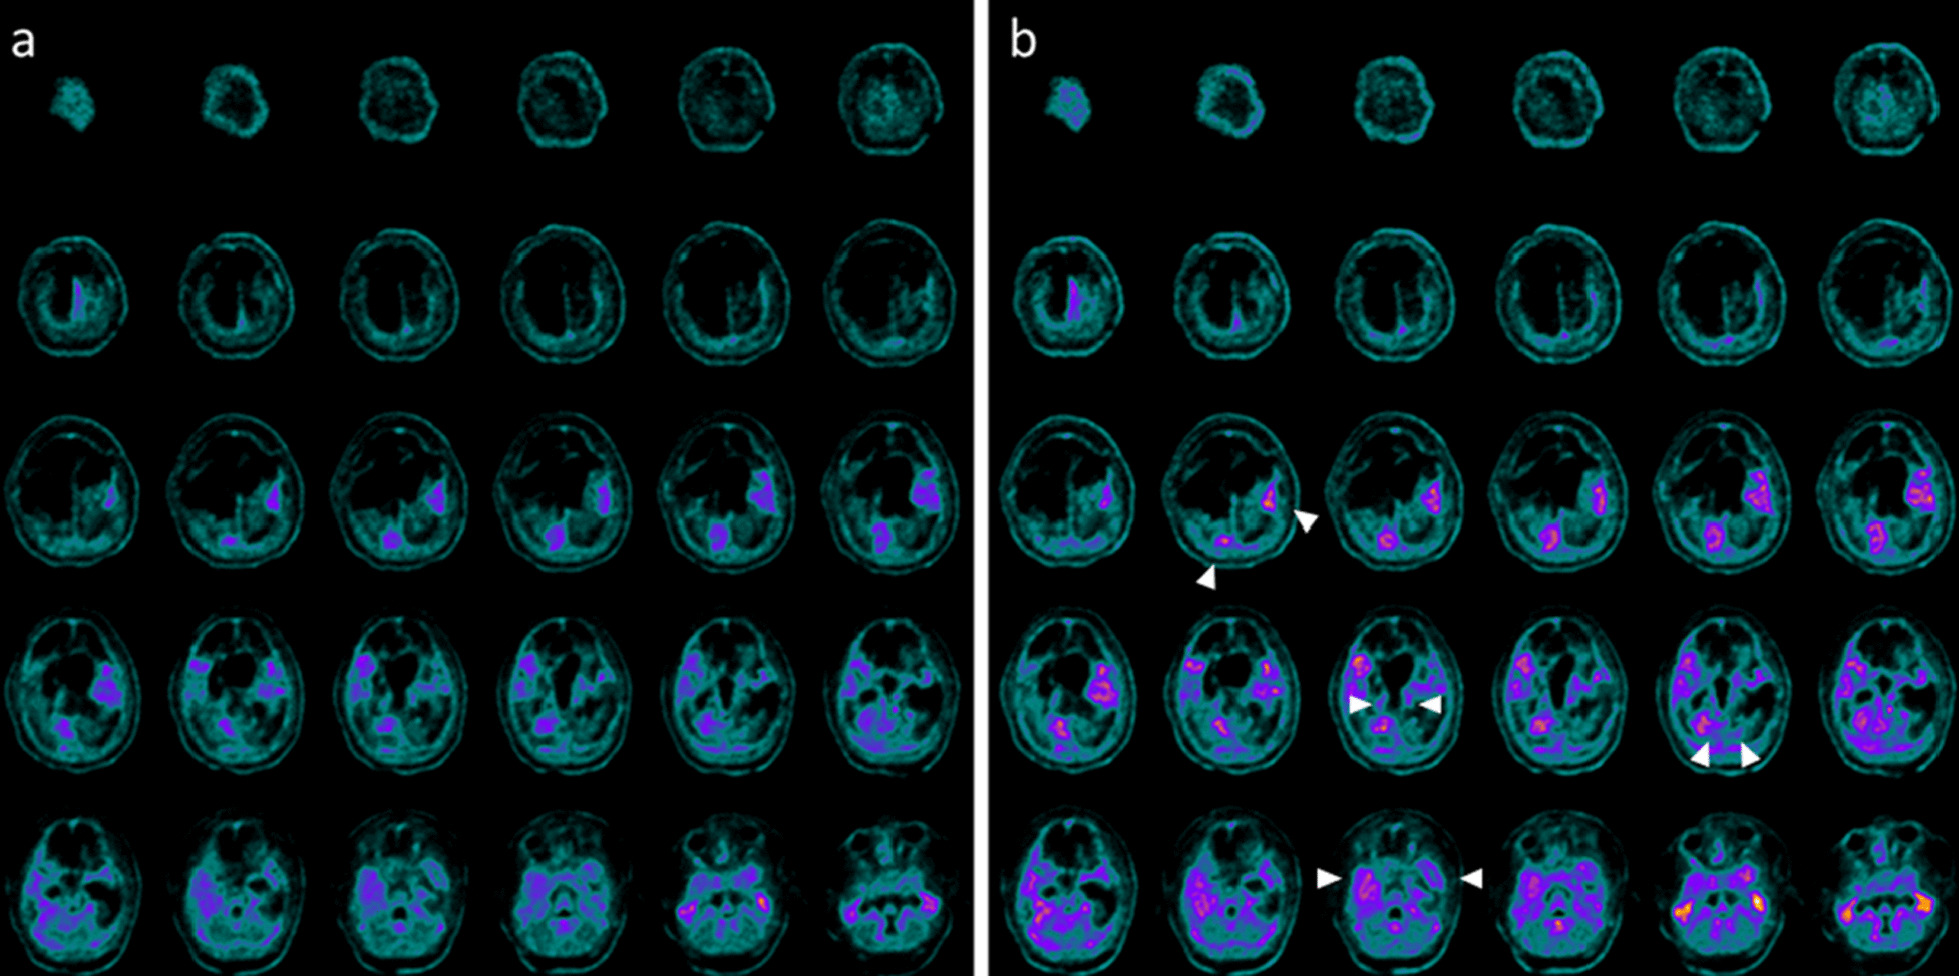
PET before and after the treatment with hr-NGF. a, b: Brain 18F-FDG PET axial slices performed before (a) and after b hr-NGF treatment. A severe global reduction in 18F-FDG uptake was observed in all cortical and subcortical regions as well as in the cerebellum (a). After hr-NGF administration, an increase in radiotracer uptake was detected in the left and right temporal cortex (right: + 18%; left: + 15%), bilateral parietal cortex (right: + 18%; left + 15%), right and left occipital cortex (right: + 10%; left: + 13%) and right and left thalamus (+ 7% and + 8%, respectively) (b)
